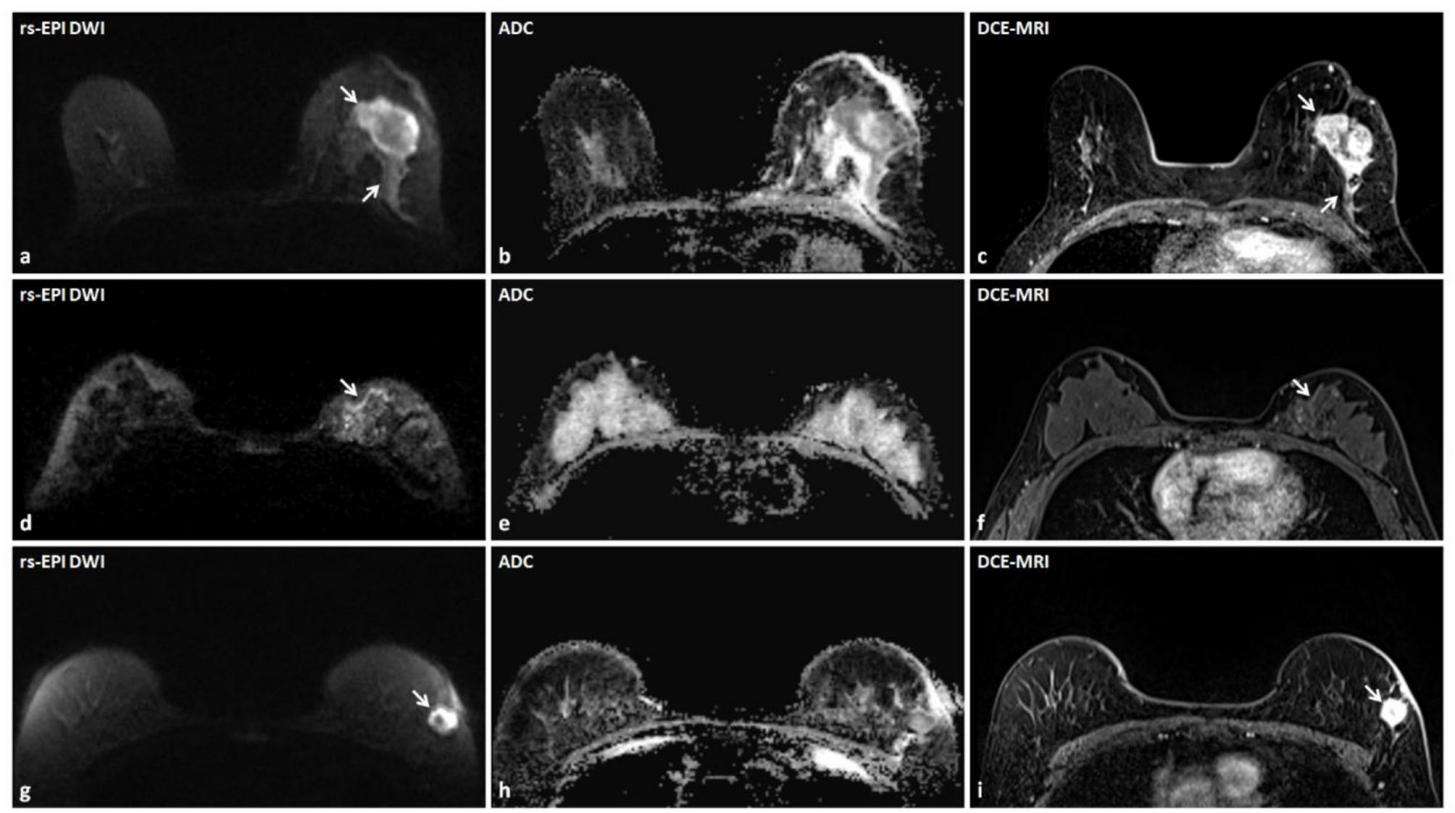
Three lesions accurately detected by rs-EPI DWI with detailed morphology characteristics in three patients. (a–c) rs-EPI DWI (*b*-value, 1,000 s/mm^2^), ADC map, and DCE-MRI of a 60-year-old woman with the left breast invasive carcinoma. (a) rs-EPI DWI shows an irregular mass (arrow) with markedly low signal on ADC map (b), and the lesion shape and extent are consistent with that delineated on DCE-MRI (arrow) (c). (d–f) rs-EPI DWI (*b*-value, 1,000 s/mm^2^), ADC map, and DCE-MRI of a 50-year-old woman with the left breast ductal carcinoma *in situ*. (d) rs-EPI DWI shows abnormal linear hyper-intensity distributed along the duct (arrow) with superior visualization than observed on DCE-MRI (arrow) (f), which may reflect the distribution of ductal carcinoma *in situ*. (g–i) rs-EPI DWI (*b*-value, 1,000 s/mm^2^), ADC map, and DCE-MRI of a 57-year-old woman with the left breast invasive carcinoma. (g) rs-EPI DWI shows a mass with the heterogeneous internal structure (arrow) and low signal in the rim on ADC map (h). (i) DCE-MRI shows an irregular mass (arrow) with heterogeneous enhancement.
